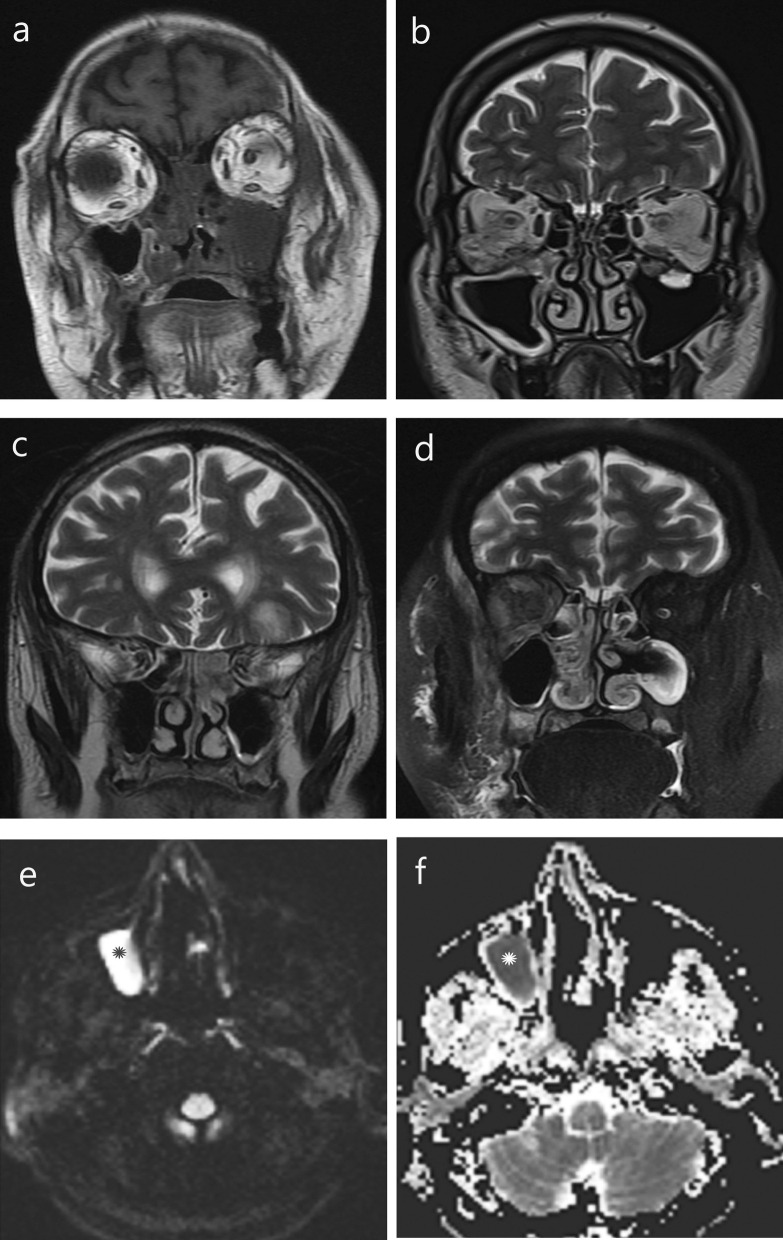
Magnetic resonance imaging findings in mucormycosis. a T1 weighted images showing hypointense polypoidal soft tissue filling bilateral ethmoids and left maxillary antrum with non-visualized left middle and inferior turbinates.T2 weighted images show b hyperintense mucosa involving bilateral maxillary sinuses with extensive inflammation in right intra- and extraconal orbital fat, c isointense soft tissue in bilateral ethmoids, d T2 fat suppressed images show hypointense mucosa in right ethmoid and nasal cavity, e Diffusion weighted images show high signal intensity in right maxillary sinus and f hypointensity in corresponding area (*) on ADC maps suggesting restricted diffusion
